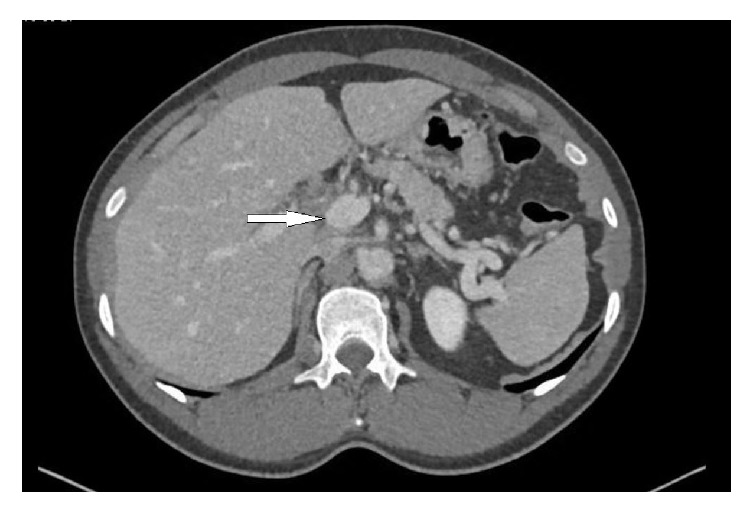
Contrast-enhanced CT abdomen after 6 months of anticoagulation showing complete recanalization of previously seen portomesenteric thrombosis.
